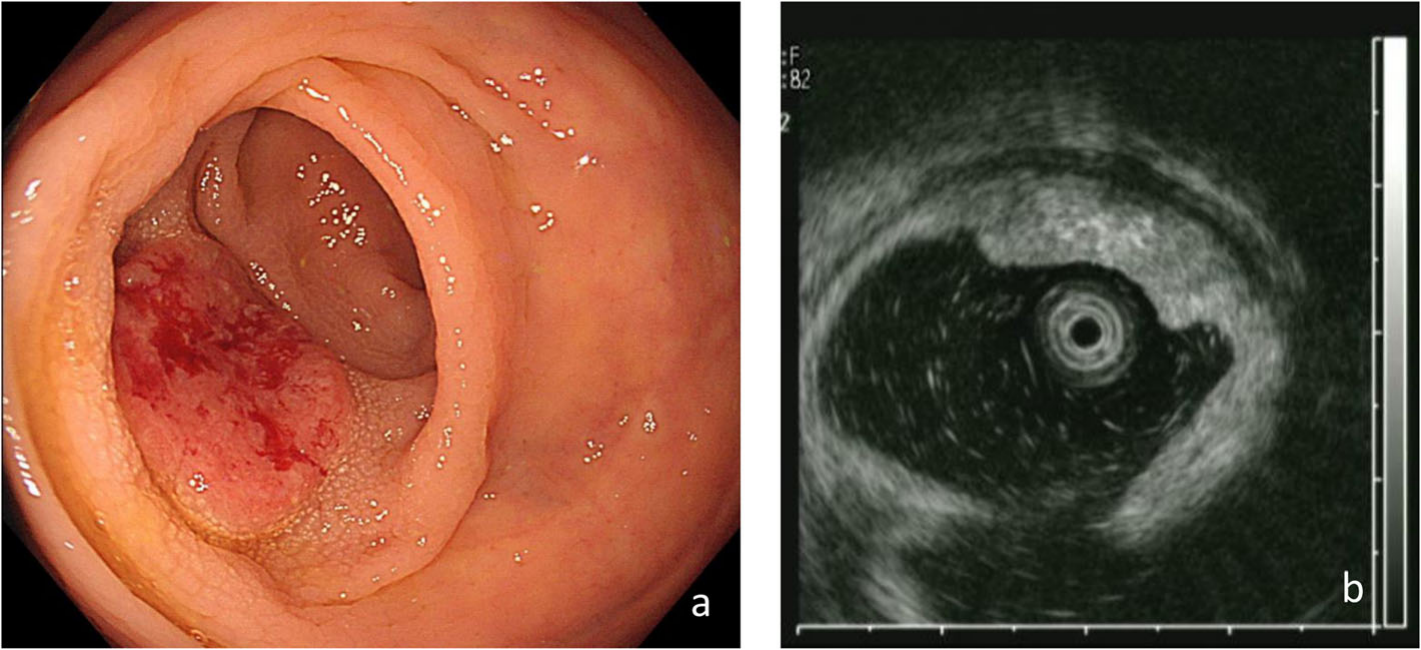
a Colonoendoscopy image revealing a rectal tumor at the left wall of lower rectum. b Colonoendoscopic ultrasonic view of the tumor demonstrating the presence of a invasive tumor with a high echo located in the third layer (muscular propria) of the rectum
